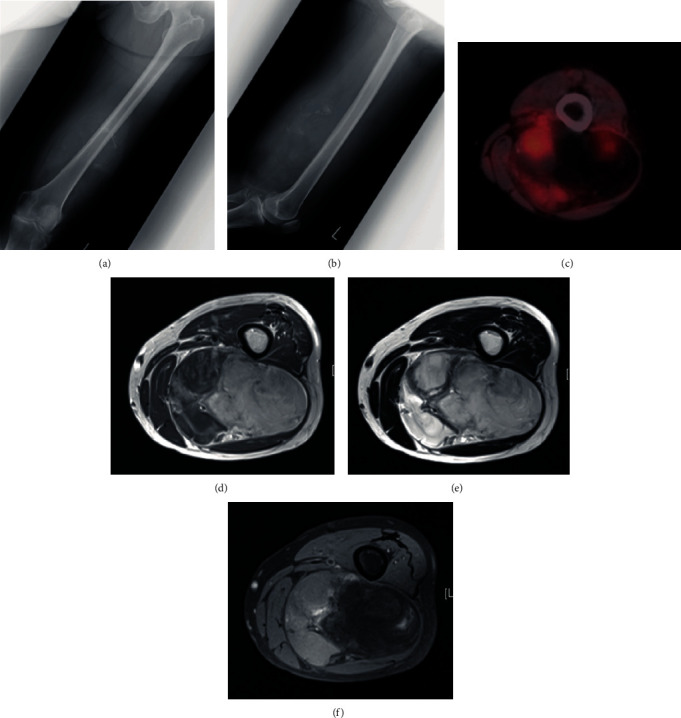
Images of myxoid liposarcoma in the left thigh at initial visit. Plain radiograph in (a) anteroposterior and (b) lateral view, (c) PET, (d) T1-weighted, (e) T2-weighted, and (f) T1-weighted fat suppressed MRI.
